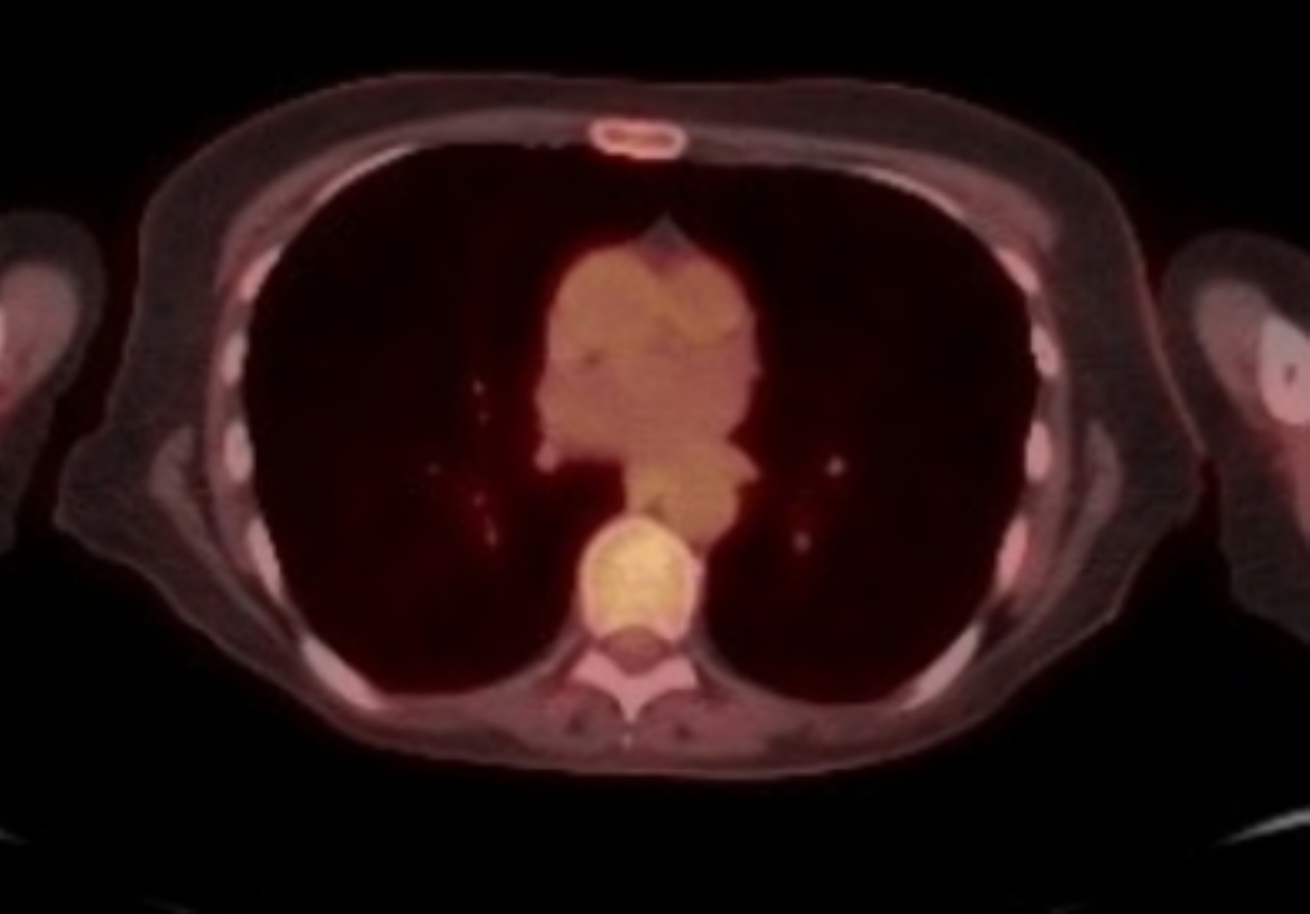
Positron emission tomography/CT (PET-CT) Subsequent and regular PET-CTs have failed to show recurrence or the development of new lesions.
